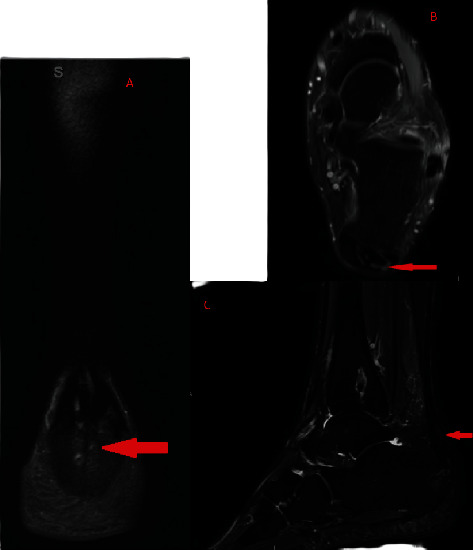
T2 fat suppression MRI with coronal (A), axial (B), and sagittal (C) views of the left ankle with arrows demonstrating thickening and the abnormal signal characteristic of Achilles tendinitis. Notably, there is no radiographic evidence of gouty tophi.
